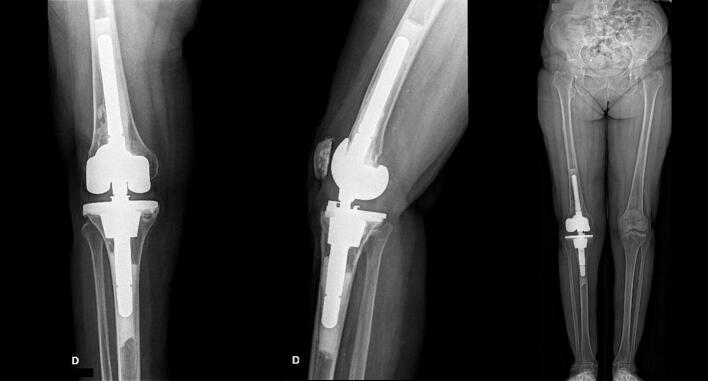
Right knee postoperative (a) anteroposterior; (b) lateral; (c) long leg view radiographs. These are demonstrating a cemented rotating-hinge TKA at 3 years post-surgery with adequate lower right limb alignment (HKA of 180°).
